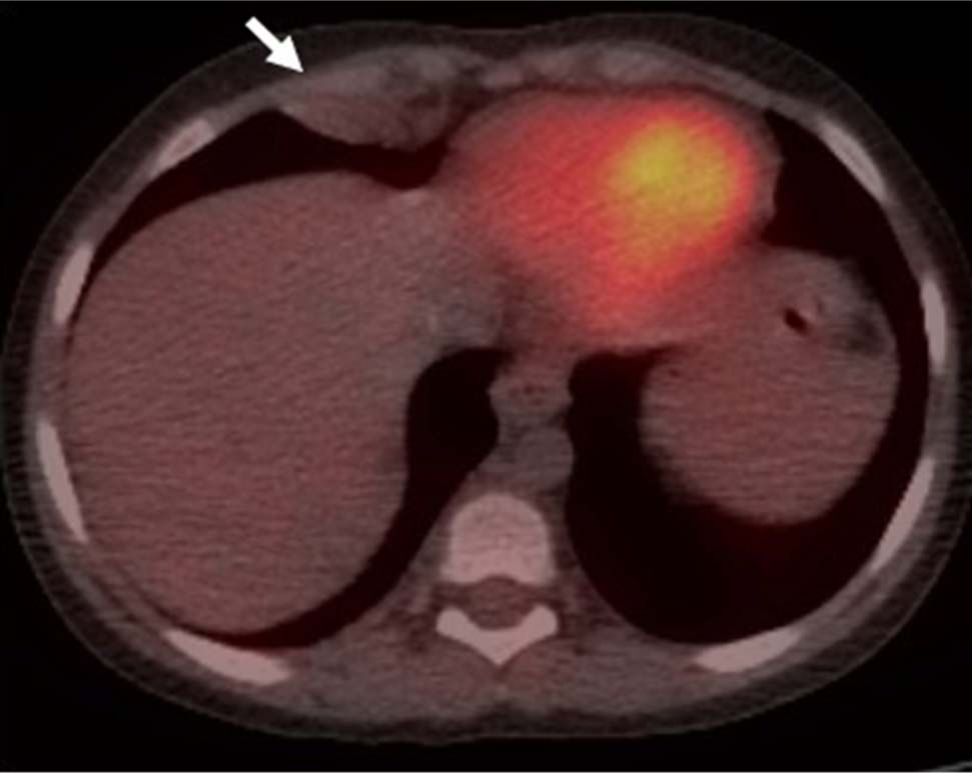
FDG PET/CT scan demonstrated interval-resolved FDG activity of the right pleural lesions 6 weeks after dose reduction of immunosuppression therapy. CT, computed tomography; PET, positron emission tomography.
